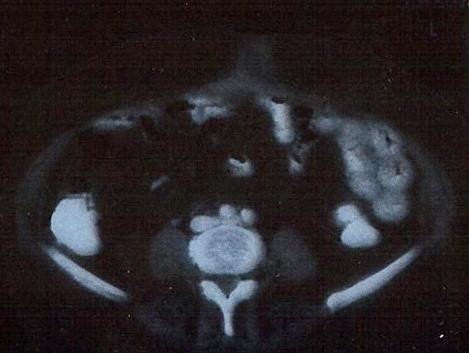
an Abdominal CT showed enhanced wall thickening in the anterior wall midline.
